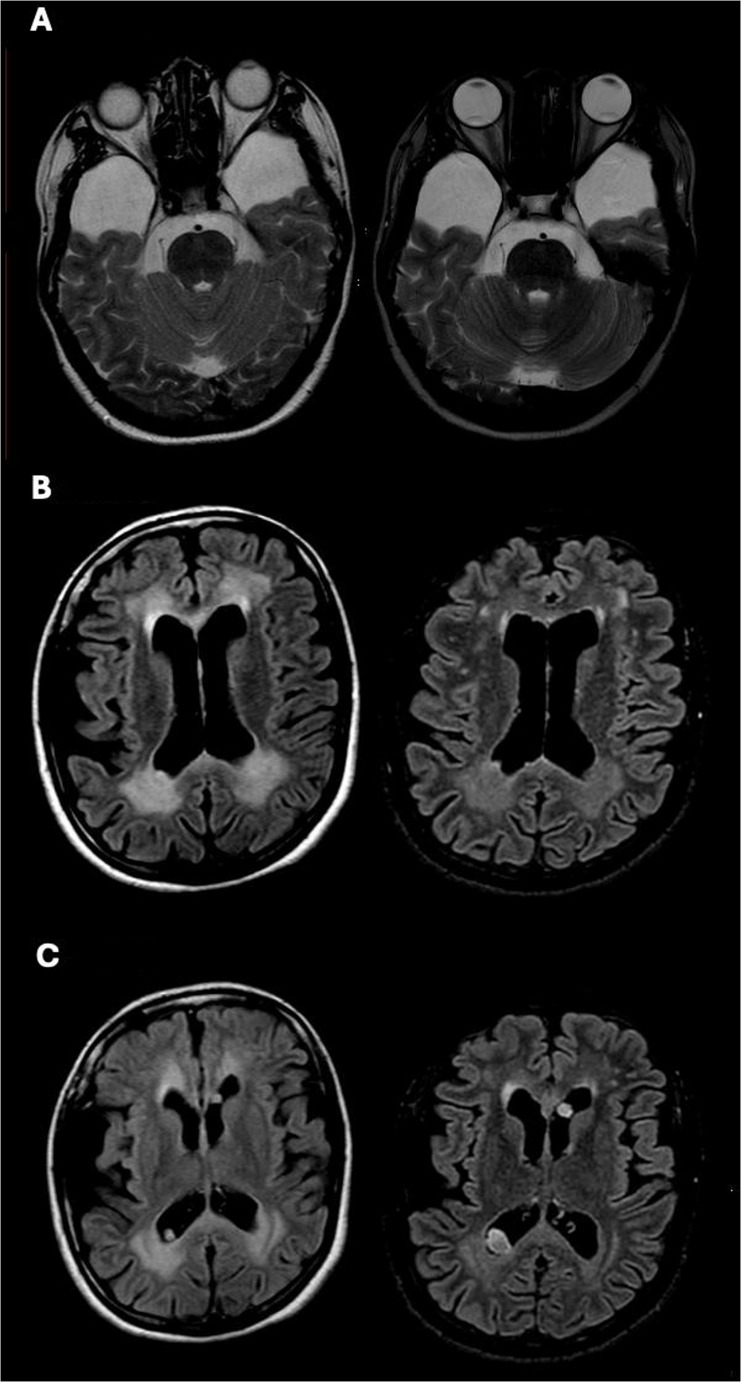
Panel (A) T2-weighted axial sections showing bilateral temporal hypoplasia. Panel (B) T2-weighted FLAIR axial sections demonstrating a reduction of altered signal in the frontal horns in the late control (right). Panel (C) T2-weighted FLAIR axial images showing two of the ten subependymal nodules, one located the right trigone (radiological convention) and one in the mid-body of the left lateral ventricle, increasing in size at the second control (right)
